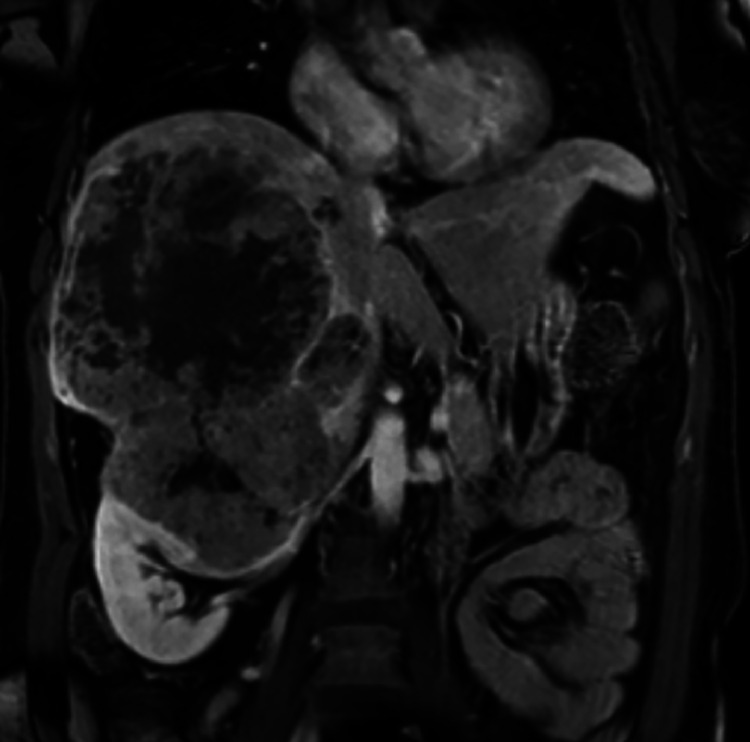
Initial T1-weighted postcontrast MRI demonstrated a 15 cm centrally necrotic HCC within the right hepatic lobe with invasion of the right upper renal pole and suspected invasion of the right adrenal gland.
